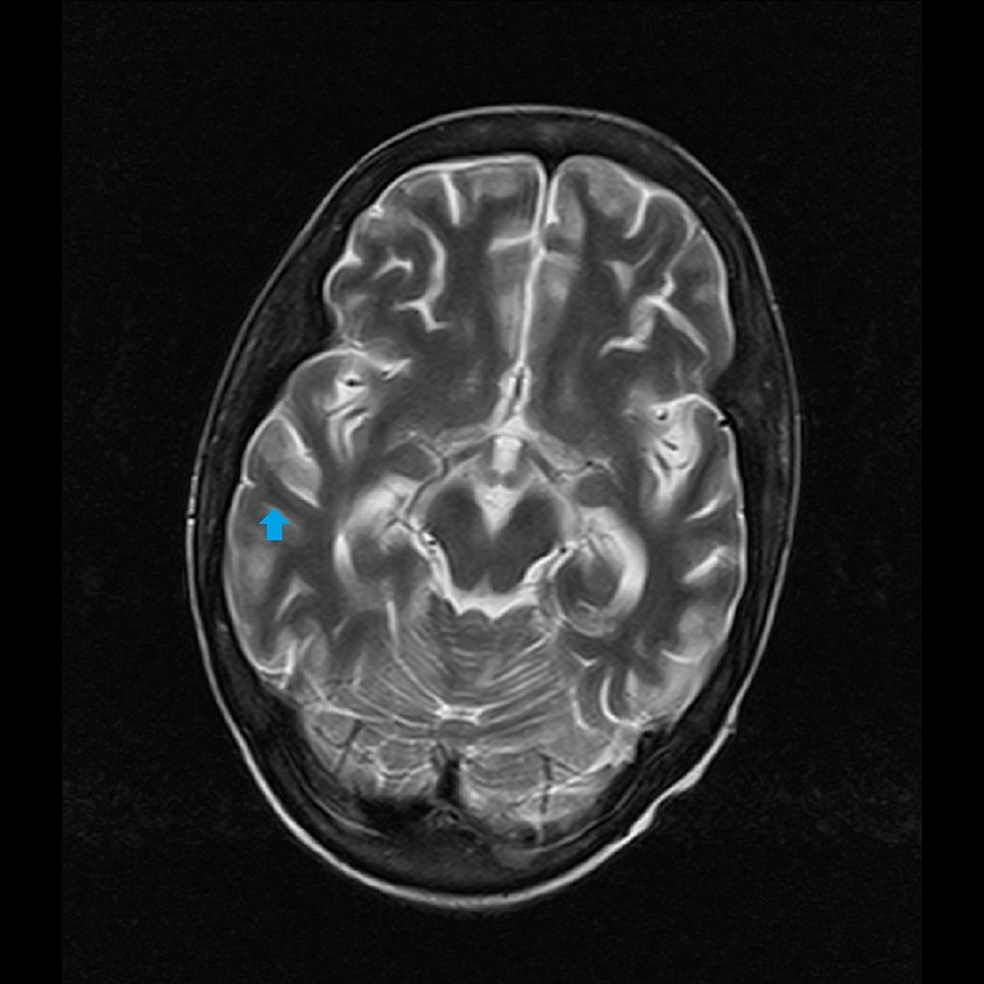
T2-weighted MRI of the transverse section of the brain showing enhanced brain parenchyma (arrow) MRI: magnetic resonance imaging
